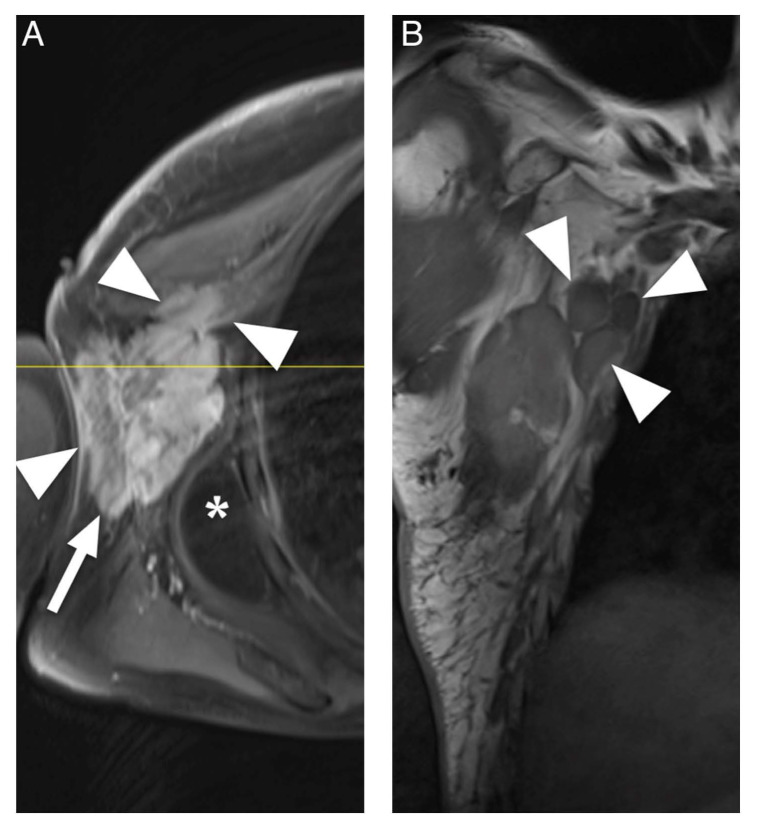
MRI of a 67-year-old man presenting with a predominantly subcutaneous manifestation of non-Hodgkin lymphoma in the right axilla. (A). Transversal contrast enhanced fat-saturated T1-weighted image shows a lobulated tumor (arrow) with inhomogeneous enhancement pattern which infiltrates into the pectoralis major and minor muscles and skin (arrowheads). Moreover, an accompanying lipoma can be noted (asterisk). (B). Coronal T1-weighted image shows associated lymphadenopathy (arrowheads).
